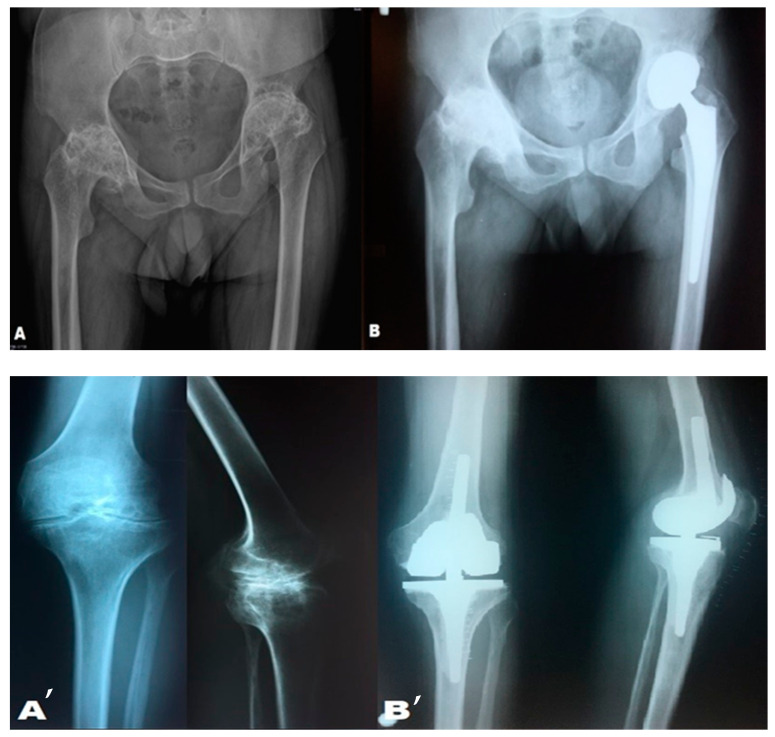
(A,B,A’,B’) X-rays of the pelvis and knee; anteroposterior and lateral views. Preoperative and postoperative X-rays (after prosthetic implantation) in patients with severe hemophilia and present inhibitors.
